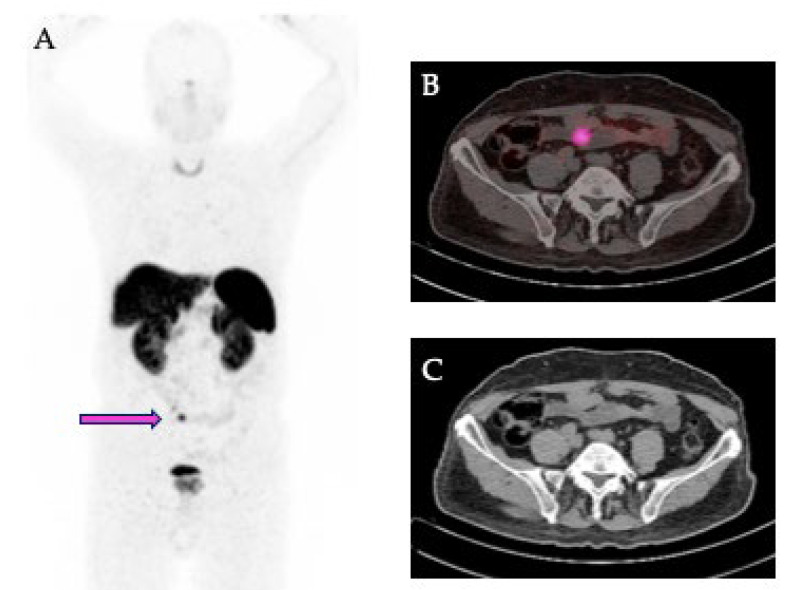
Representative images of a 68Ga-DOTATOC PET/CT scan in a patient with NET of the ileum. (A) Maximal intensity projection view showing the primary tumor in the ileum (pink arrow). (B) Transaxial fusion image showing the segmentation (pink) of the primary tumor. (C) Corresponding transaxial CT image.
